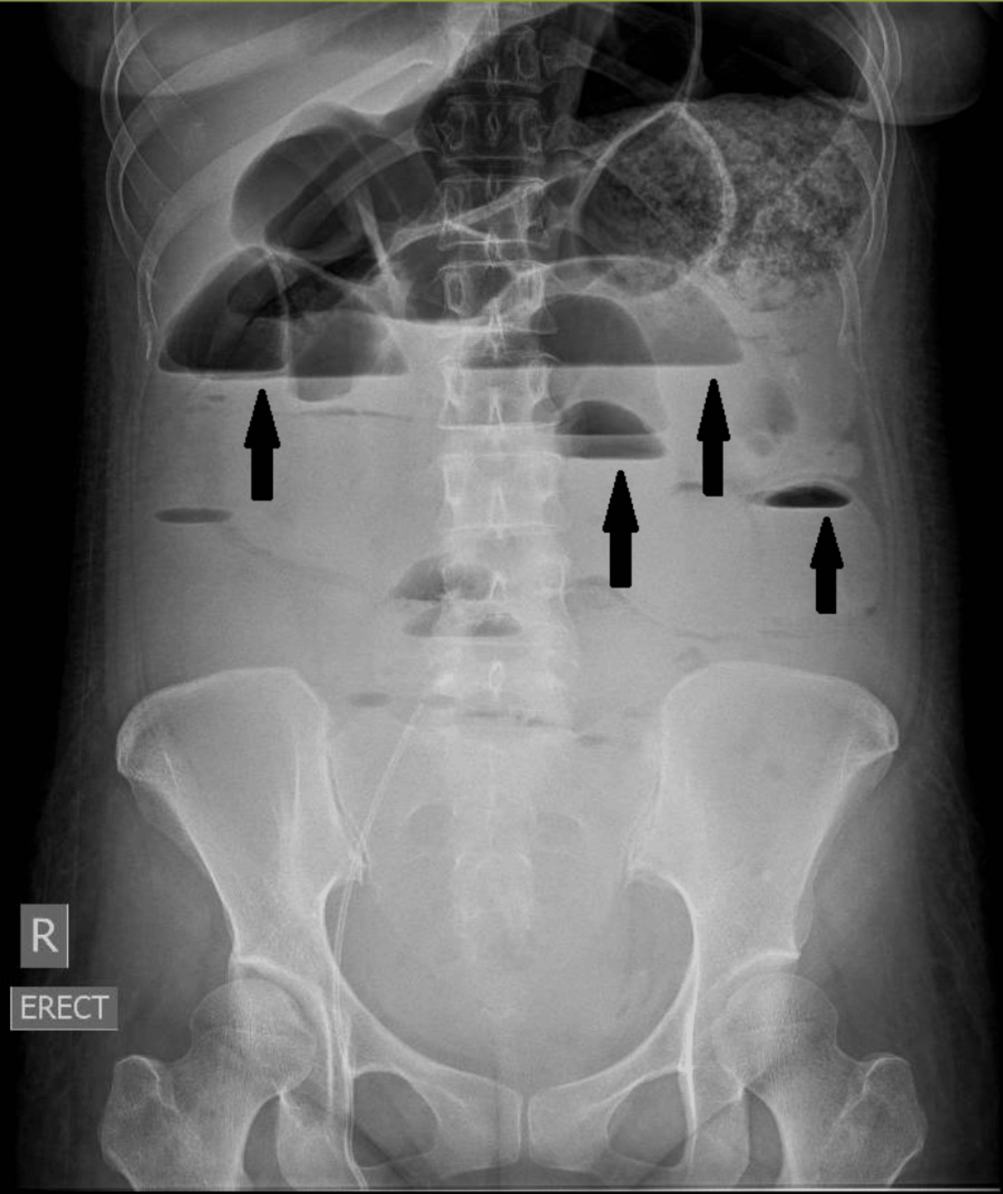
Abdominal radiograph showing air-fluid levels (black arrows) representative of IPO IPO, intestinal pseudo-obstruction
